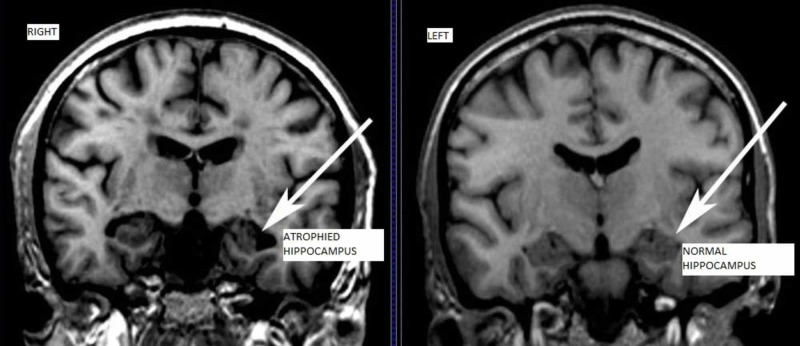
MRI showing atrophy in hippocampus indicated with arrows Arrowhead in the right image shows atrophied hippocampus in AD patient whereas the arrowhead in the left image shows normal hippocampus. AD - Alzheimer's disease Recreated under creative commons license [14].
